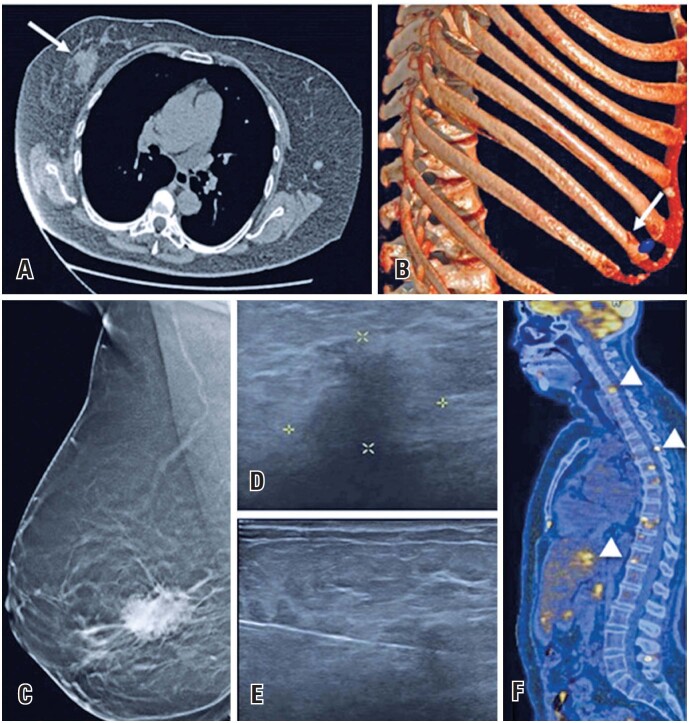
A 69-year-old woman presents to the emergency department with complaints of chest pain after a massage. (A and B) She underwent a chest computed tomography (CT), which revealed a rib fracture and an irregular density in the right breast (arrows). (C) Further investigation with mammography demonstrated an irregular spiculated mass —*BIRADS 4*. (D and E) Ultrasound-guided core biopsy revealed a *lobular carcinoma.* (F) A positron emission tomography (PET)-CT scan showed also hypermetabolic metastatic bone and liver lesions
